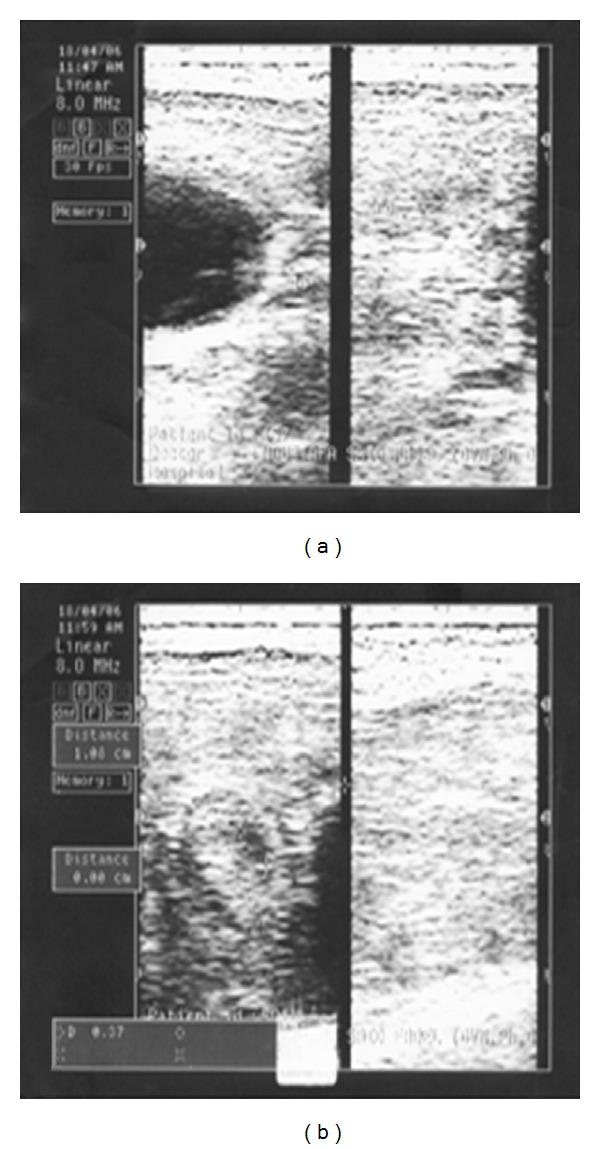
Effect of calcium soap of fatty acid supplementation on the activity of the ovaries of ewes by using ultrasonography. (a) Control ewe nontreated had small and smooth ovary. (b) Calcium-soap-of-fatty-acid-treated ewe had normal-sized ovary with follicles.
